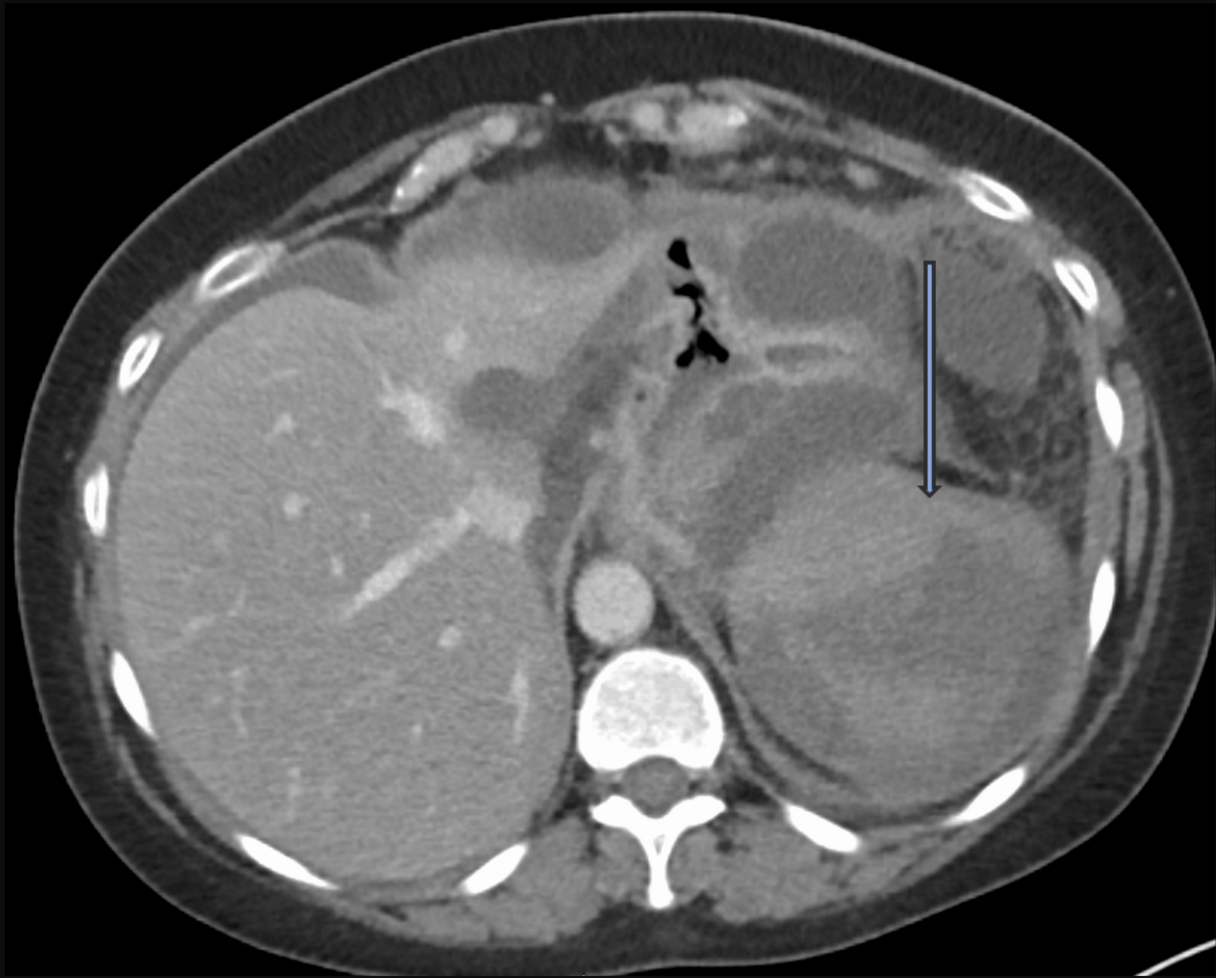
CT scan of the abdomen demonstrating spontaneous splenic rupture with a large subcapsular hematoma, perisplenic hematoma, and hemoperitoneum The blue arrow points at the large subcapsular hematoma CT: computed tomography
